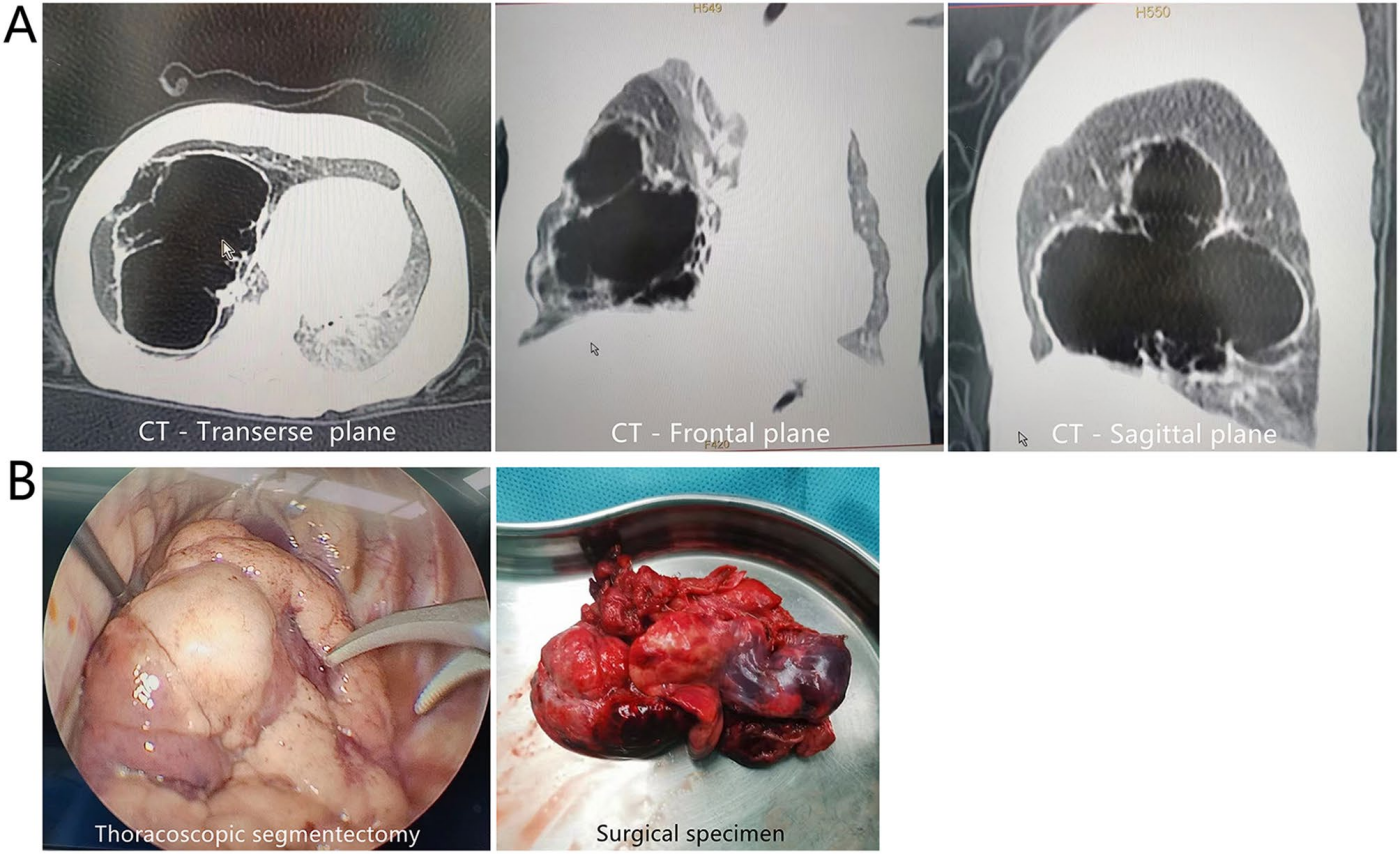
In case 4, a 3-month-old male infant were diagnosed with a suspected right-sided CPAM based on an abnormal 20-week prenatal ultrasound. A chest computed tomography (CT) scan was performed prior to surgery (A), revealing a massive right-sided cystic lesion measuring 63 × 52 × 49 mm, along with poor ventilation of the remaining lungs. The patient underwent a thoracoscopic procedure, wherein the dorsal and basal segments of the right lower lobe were excised (B).
